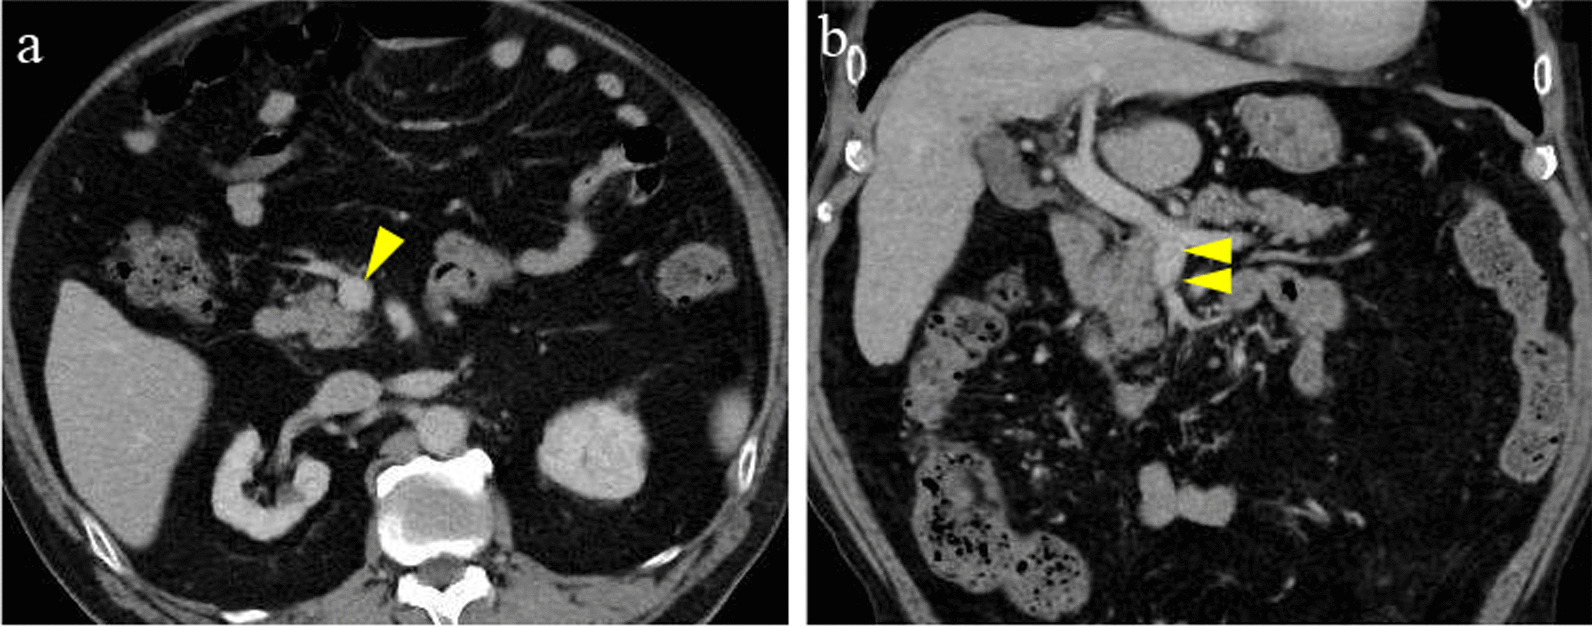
Abdominal computed tomography at 45 days after discharge. The SMV thrombosis has disappeared almost completely (yellow arrows). Axial image (a). Coronal image (b)
